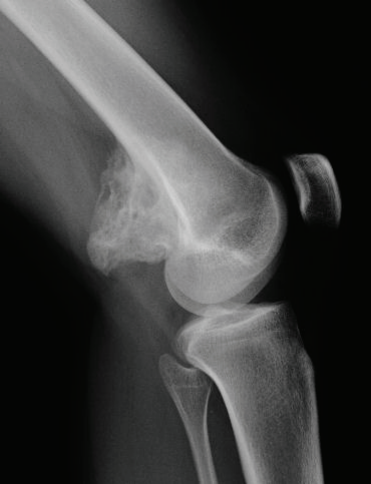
Case 1 - lateral view. Radiographs demonstrate a sessile ossified mass arising from the posterior surface of the left distal femur metaphysis. No cleavage plane is observed.
